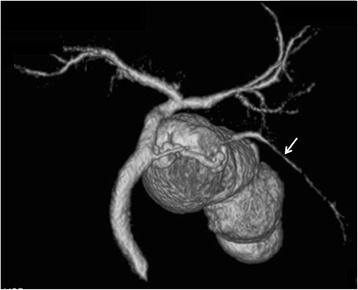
Three-dimensional DIC-CT image (dorsal view) showing a type II accessory hepatic duct. The accessory hepatic duct drained segment 6 (*arrow*) and was classified as belonging to the posterior branch type
